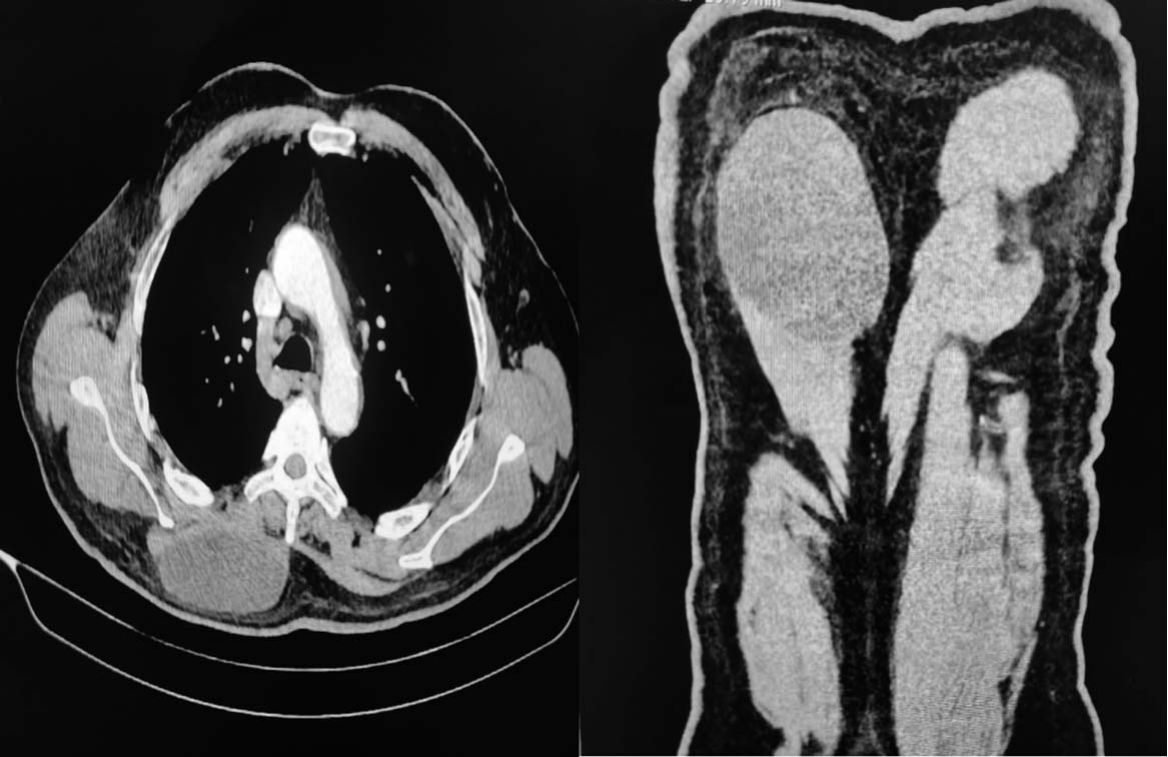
Computed tomography scan of the chest with intravenous contrast shows a fluid density cystic area measuring about 9.8×8.8×3.8 cm (LS×TS×AP) (length, transverse, and anteroposterior) involving the subcutaneous tissue of the right posterior chest wall extending up to the midline. It is associated with mild adjacent fat streaking. It appears inseparable from underlying posterior spinal muscles.
